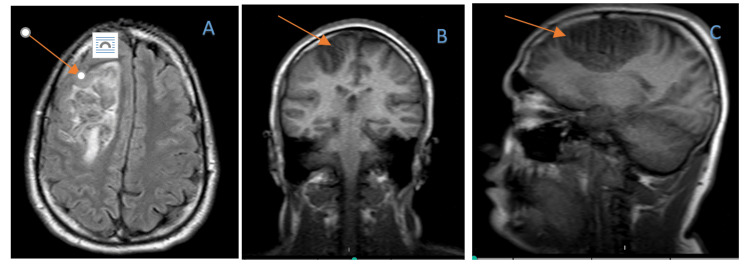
MRI of the brain showing the area of signal abnormality in the frontal lobe with regional mass effect with concern for hemorrhagic infarct A: axial view; B: coronal view; C: sagittal view
